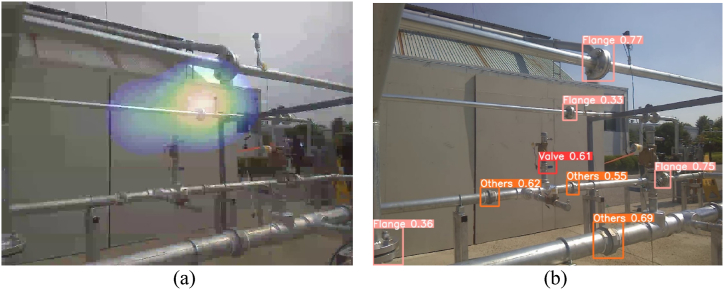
(a) The ultrasonic camera detects both the actual leakage and its reflection, as if there are two leaks. (b) Pipe joint detection result from the image taken by the ultrasonic camera.
